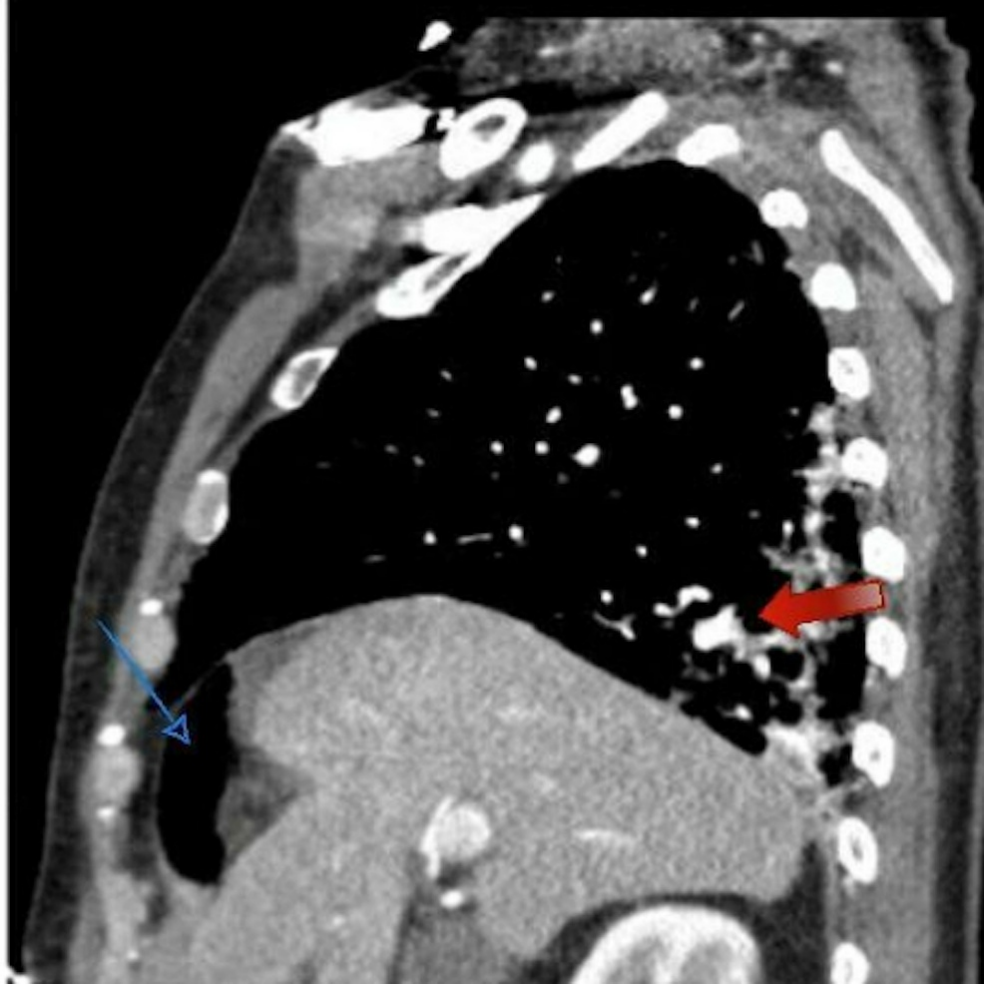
Chest CT without contrast sagittal view showing moderate patchy bilateral airspace disease most pronounced at the lung bases with areas of bronchiectasis (red arrow), cystic changes, and pneumoperitoneum (blue arrow)
